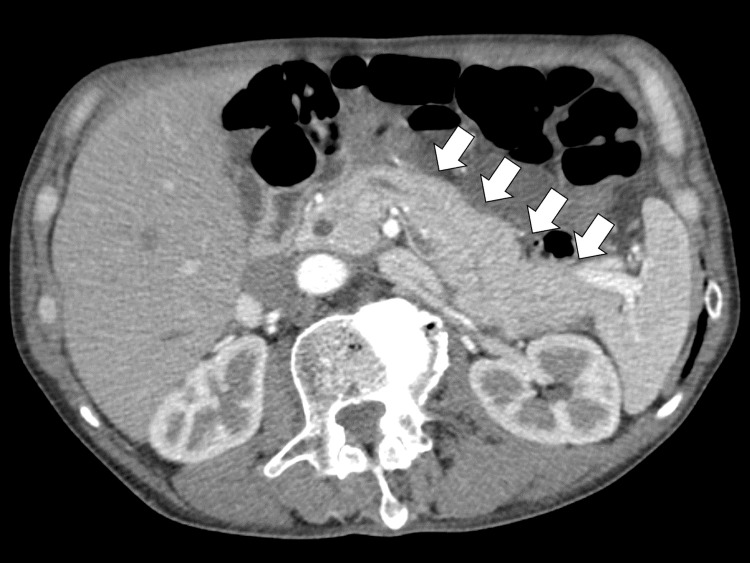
Enhanced abdominal computed tomography shows enlargement of the pancreas without masses in the pancreas and systemic lymphadenopathy (white arrows).
